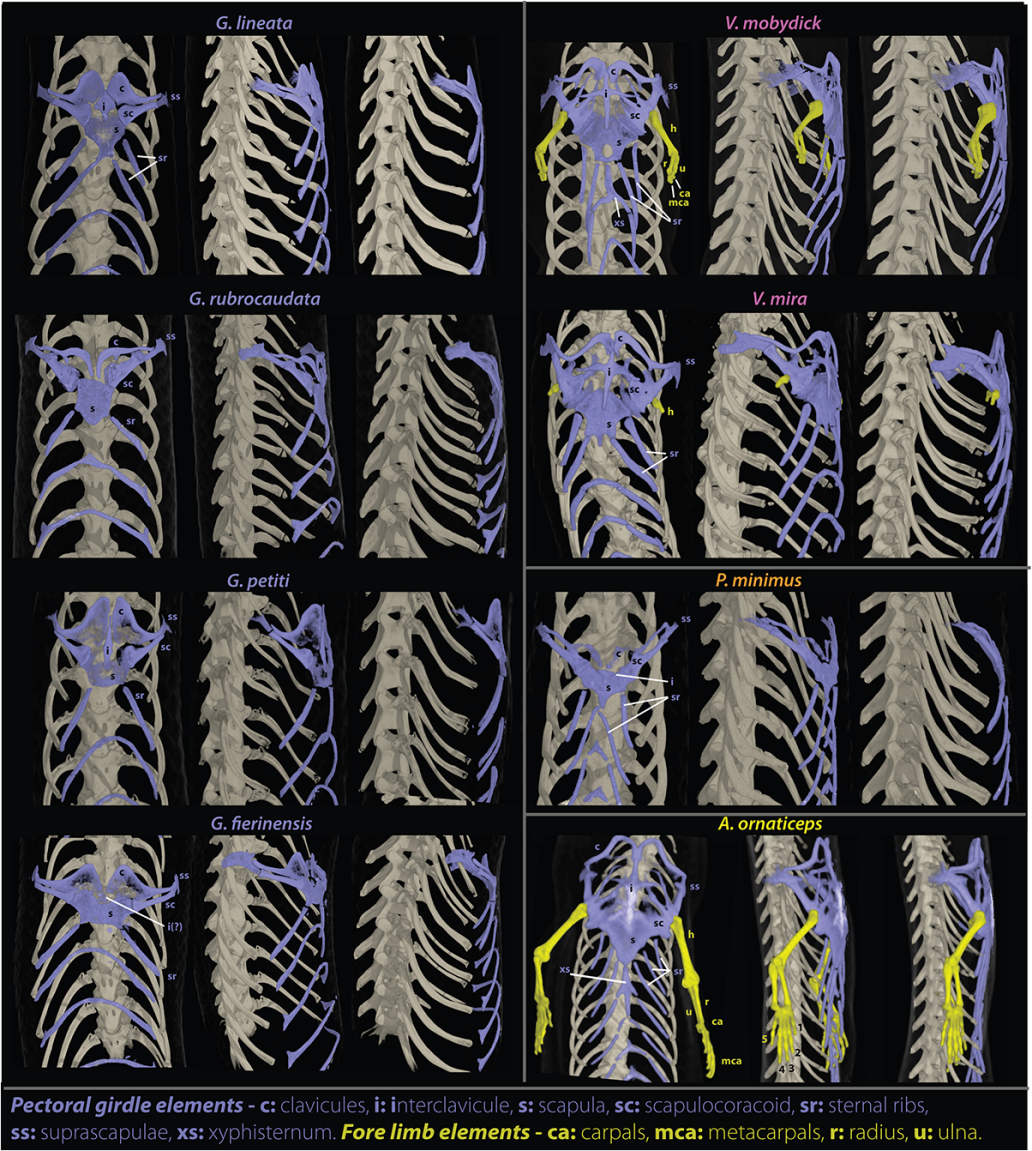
Pectoral girdle and forelimbs skeletal anatomy. Computed tomographic reconstruction of the pectoral girdle (in purple) and forelimbs (in yellow) of seven different species of blind sand-swimming skinks and one species without limb regression (*Amphiglossus ornaticeps*), based on microtomographic scans, in ventral, ventrolateral and lateral view (not to scale).
